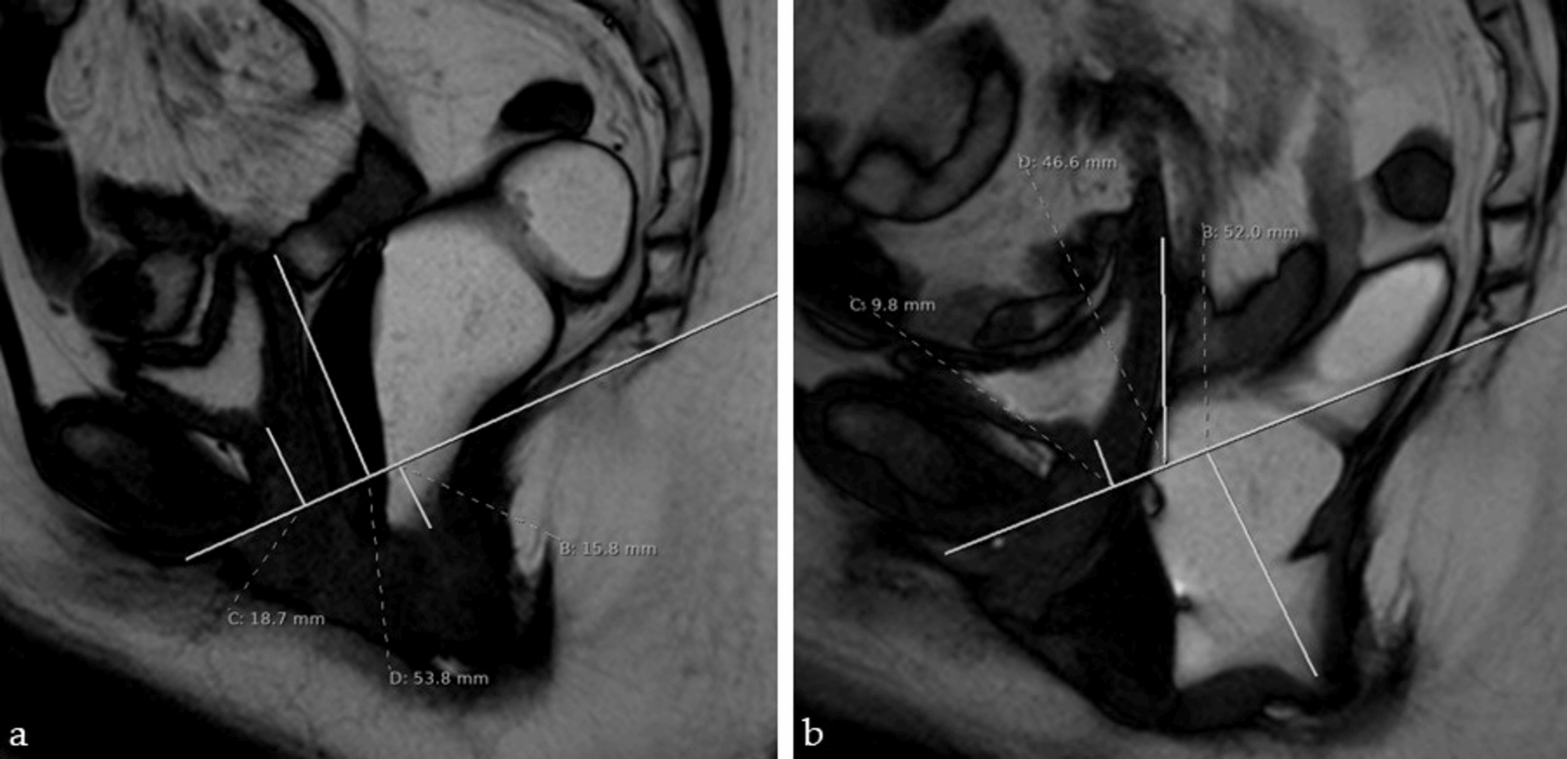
MRI defecography, sagittal view. (a) At rest, anterior, middle, and posterior compartment descent measured relative to the pubococcygeal line (PCL): 1.9 cm, 3.4 cm, and 1.6 cm, respectively, demonstrating mild posterior descent. (b) During evacuation, descent below the PCL increases to 1.0 cm (anterior), 4.7 cm (middle), and 5.2 cm (posterior), revealing marked posterior compartment collapse with rectocele formation and an obstructive enterocele measuring 52.0 mm.
